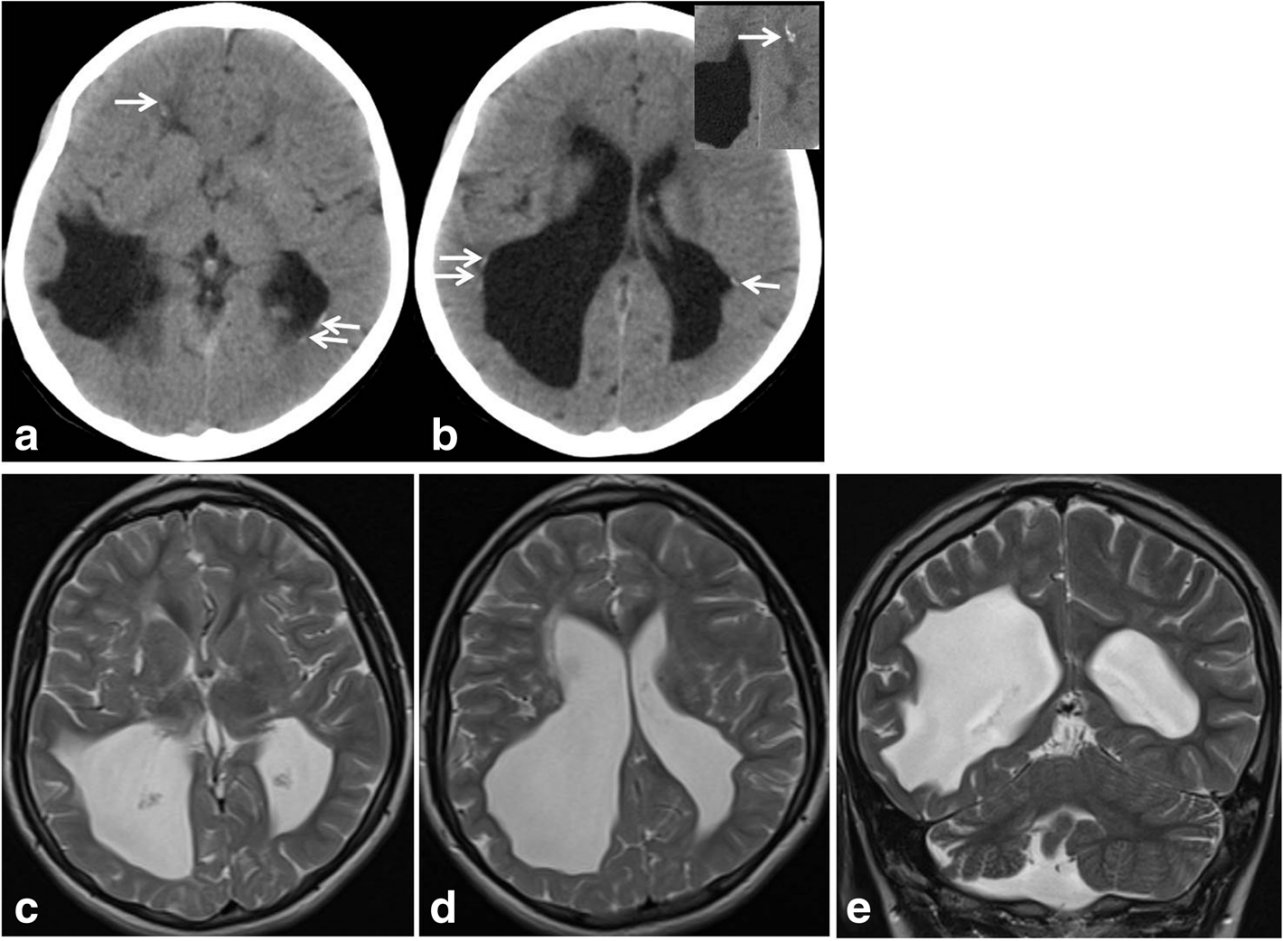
COL4A1*-related leukoencephalopathy –Brain CT, a and b and brain MRI, c-e, show «ex vacuo» enlarged lateral ventricles with irregular profiles mainly posteriorly and on the right associated with lacunar infarctions in the right basal ganglia. CT demonstrate small sub-ependymal calcification and insert in b show calcification in the sub-cortical white matter (arrows). Coronal image in E demonstrate right posterior cranial fossa and right cerebellar hemisphere hypoplasia
